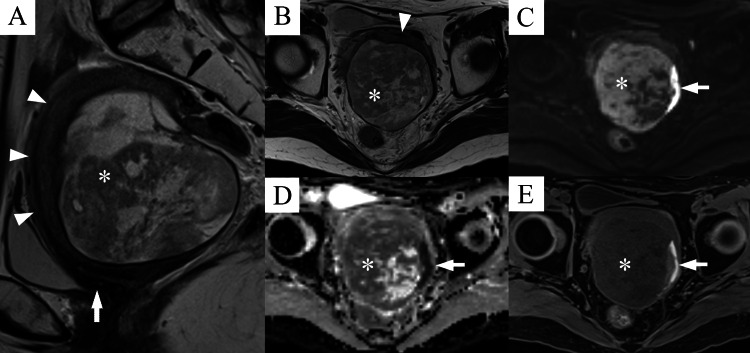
MRI findings of the tumor (A), (B) T2-weighted image of the pelvis. (A) Axial and (B) sagittal views. Arrowheads show the endometrium, and the arrow shows the uterine cervix. The tumor was located from the myometrium to the subserosa and extraserosa (asterisk). (C-E) Axial diffusion-weighted image (C), apparent diffusion coefficient (ADC) map (D), and fat-saturation T1-weighted image (E). The tumor is indicated by the asterisk. Hemorrhage was identified (arrow). (C) and (D) show heterogeneous diffusion restrictions.
